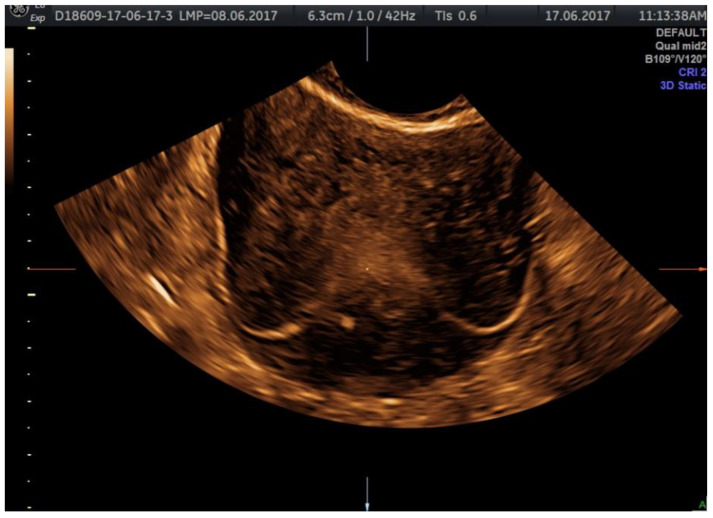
3D-ultrasonography static mode HyFoSy: the intramyometrial segment of the fallopian tubes.
